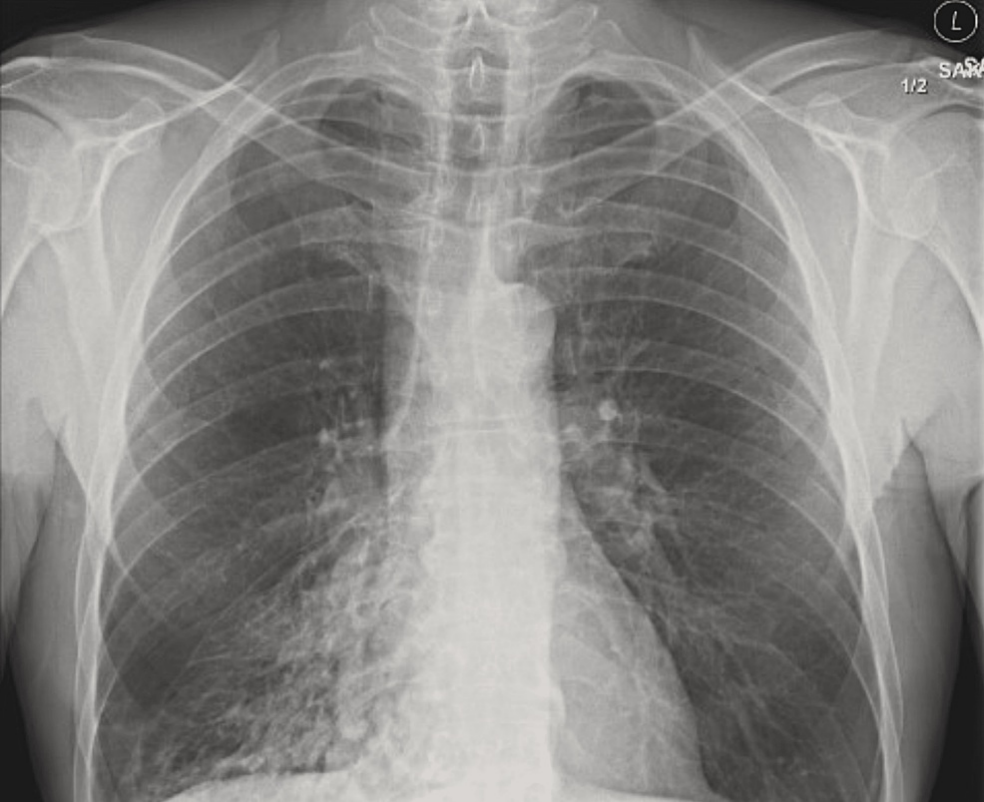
Chest X-ray showing patchy areas of consolidation on medial right lower lobe
